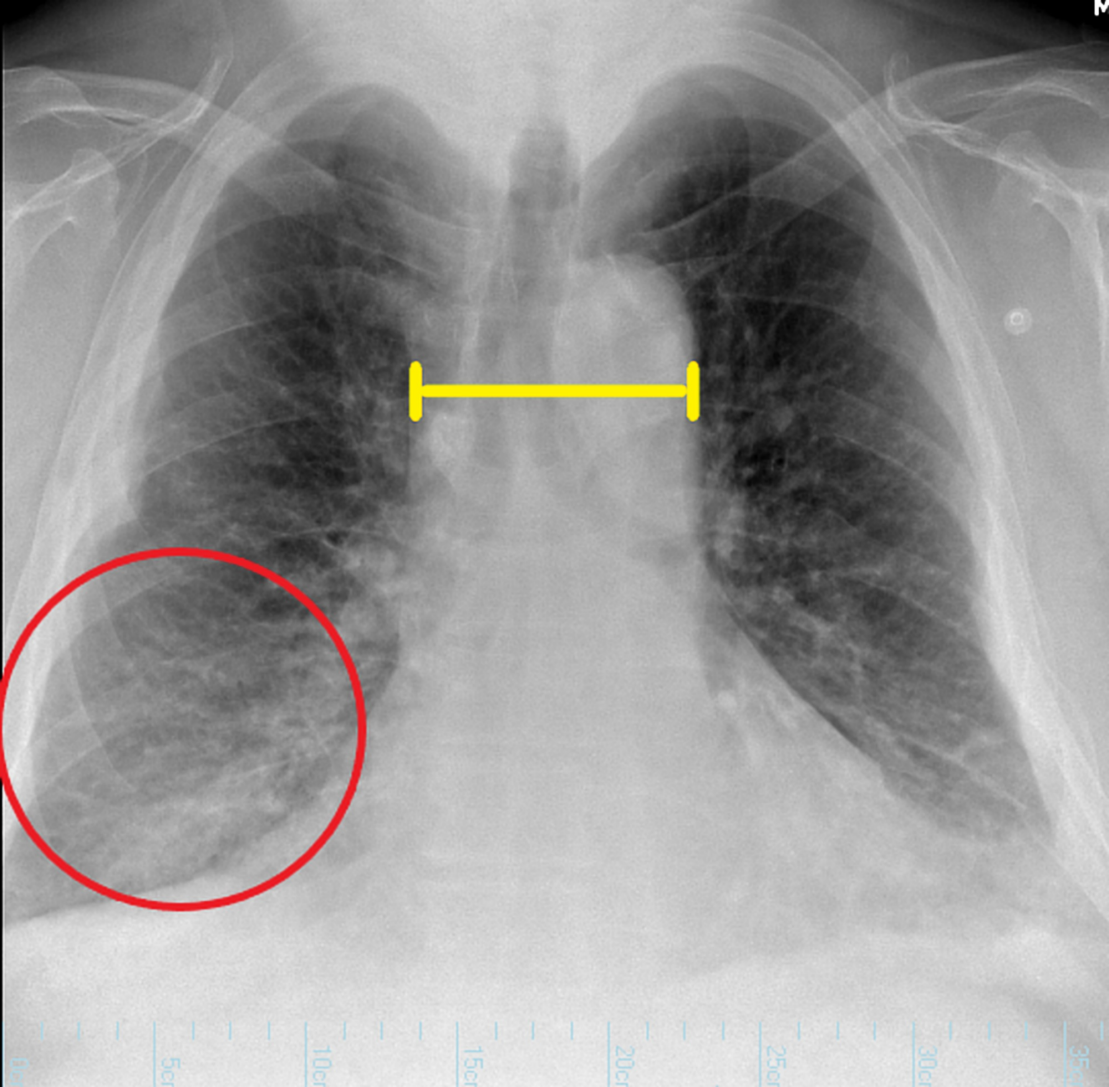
Chest X-ray, showing a right-sided lobar consolidation (red circle) and a widened mediastinum (yellow bar).
